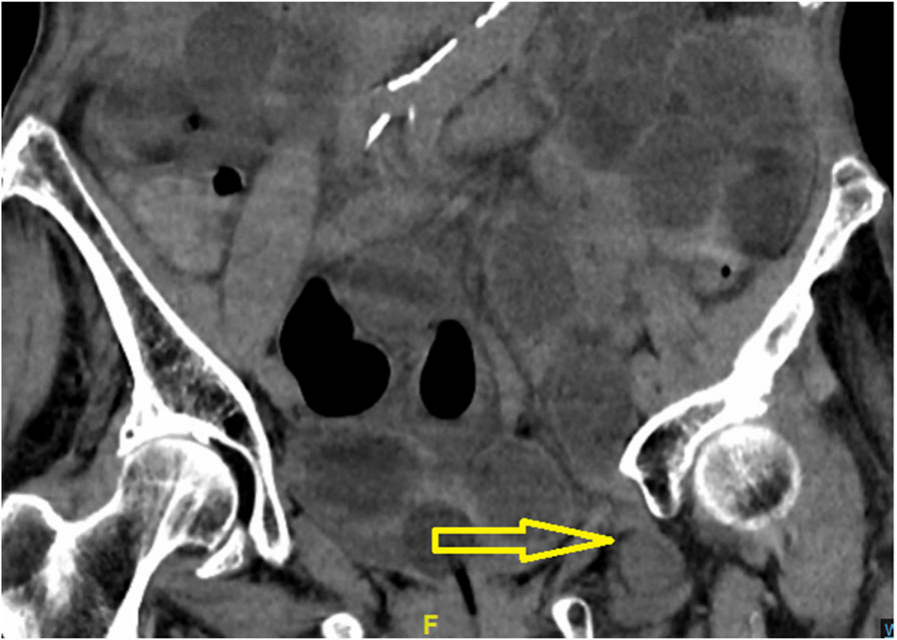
Coronary CT scan. The yellow arrow indicates bowel in the obturator foramen
